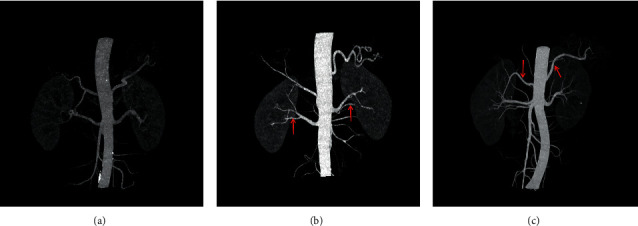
Types of renal artery and accessory renal artery described in enhanced renal CT scan. Different accessory renal arteries with renal CT scan. (a) No accessory renal artery (NAR); (b) main renal artery branches outside the renal hilum (MRAB); (c) the accessory renal artery (ARA).
